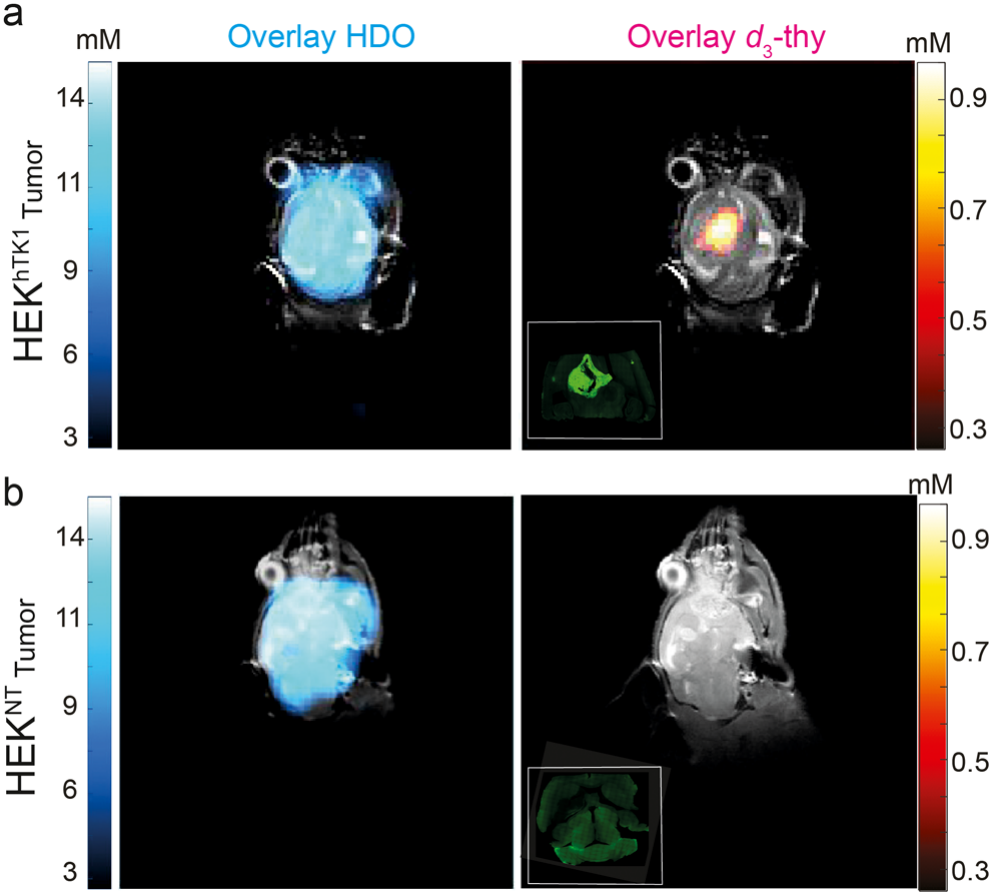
In vivo*^2^H MRI mapping of hTK1 reporter
